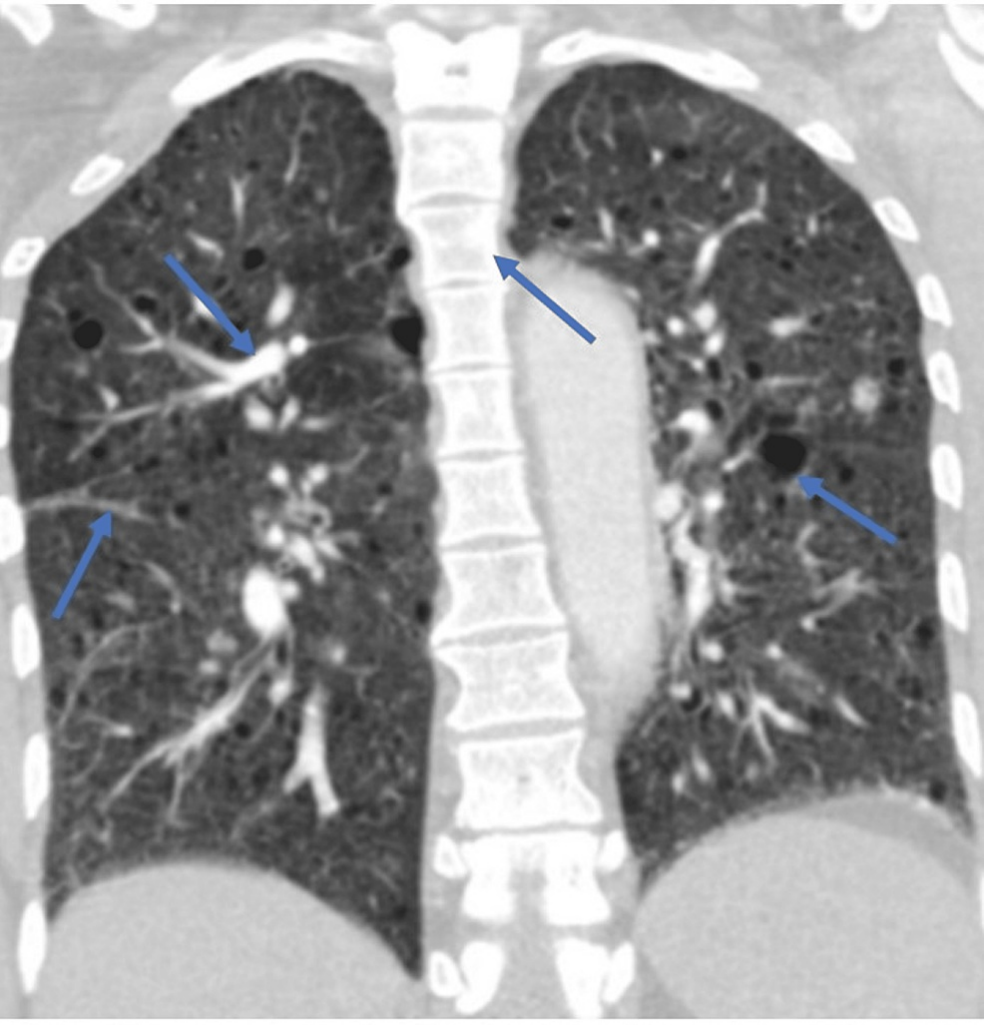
CT chest showing multiple pulmonary nodules, ill-defined opacities, numerous thin-walled parenchymal cysts and sclerotic lesions of the vertebral bodies (blue arrows). CT: computed tomography.
